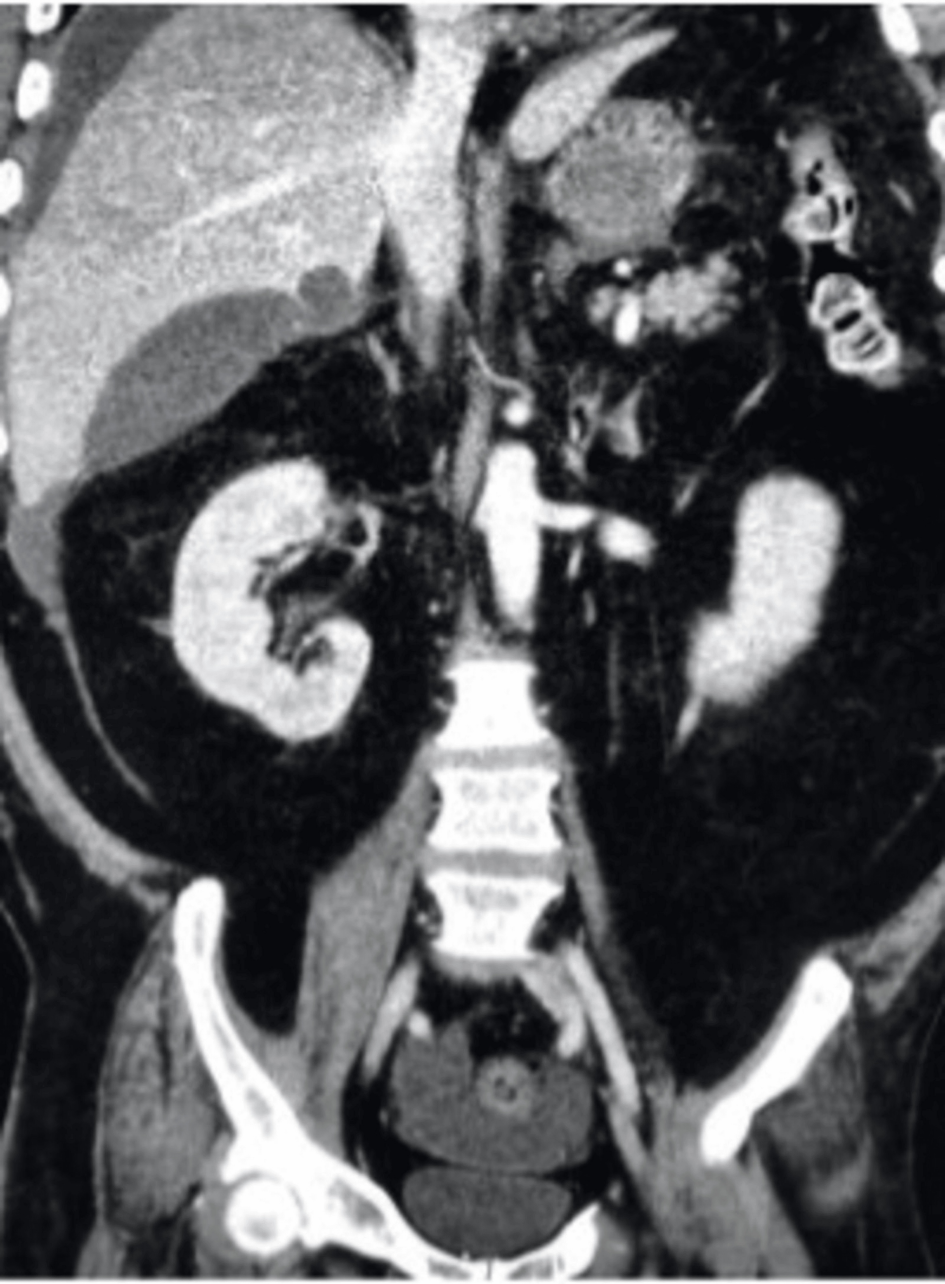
CT imaging showing a rounded or irregular low-density area (dark region) suggestive of fluid collection adjacent to the surgical site
